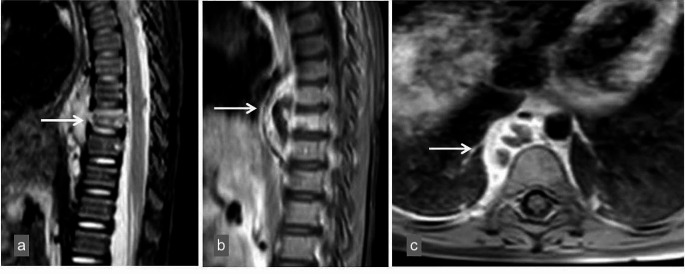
T2-weighted sagittal images (a) showed increased signal and loss of height in the 9th thoracic vertebra (arrow). Contrast-enhanced T1-weighted sagittal (b) and axial images (c) showed increased contrast enhancement in the 9th thoracic vertebra, a heterogeneous densely contrasted soft tissue lesion (abscess) with a cystic-necrotic component extending to the upper and lower adjacent prevertebral space (arrow)
